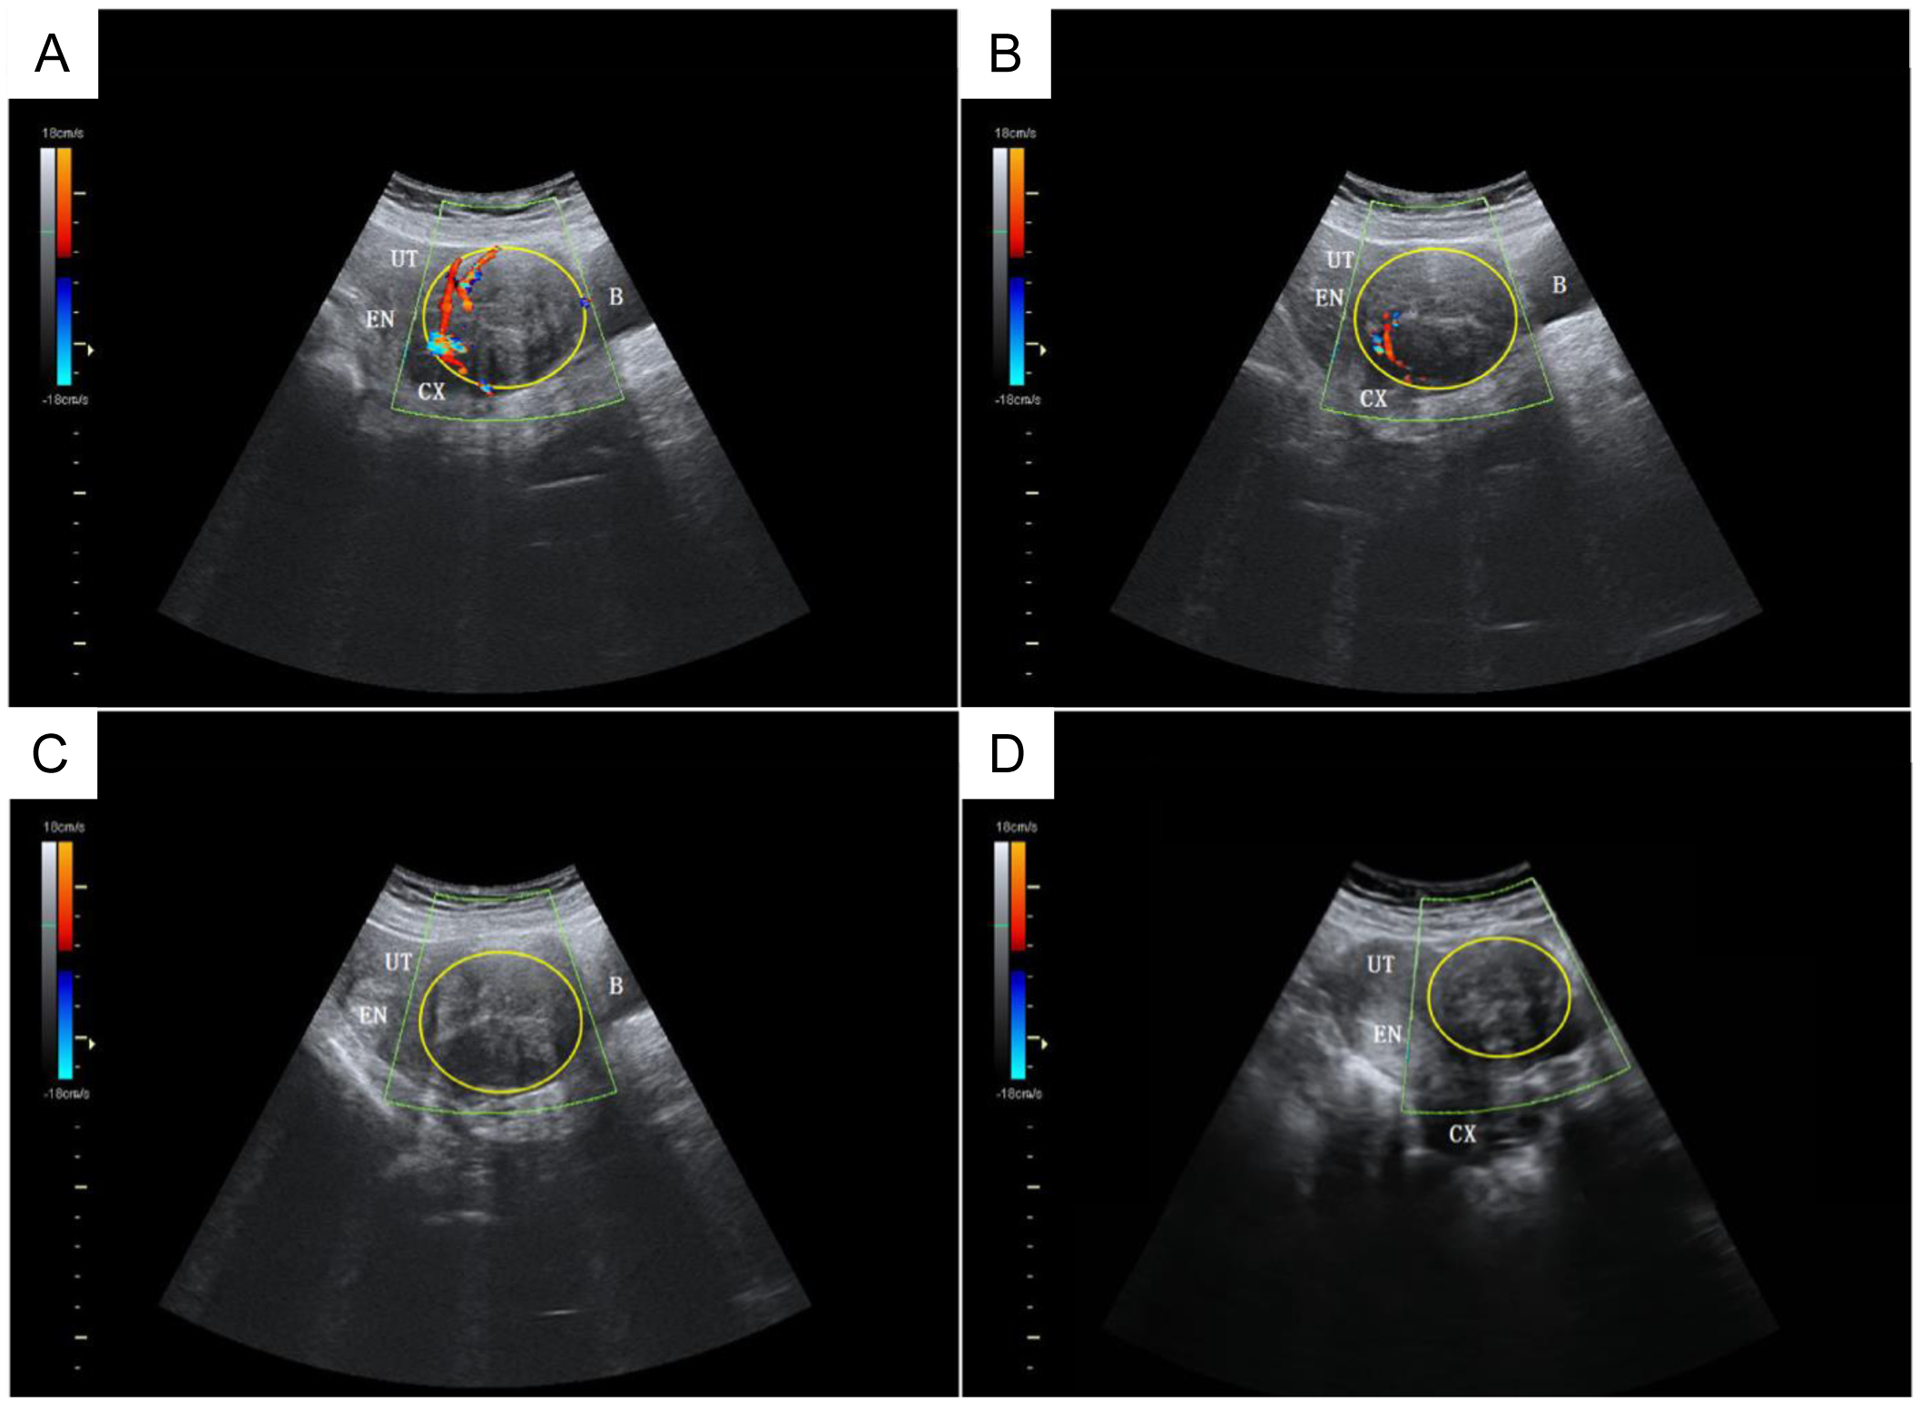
Color Doppler ultrasound imaging pre, peri, and postthermal HIFU ablation. b-mode ultrasound (US) images with color Doppler flow imaging (CDFI) from a 44-y-old patient before and after treatment with thermal HIFU. (a) Pretreatment image shows the vascularity of the fibroid for treatment. (b) Intraoperative assessment result shows that the targeted vessel in the treatment cell was not detected by CDFI and Power Doppler Imaging (PDI). (c) Posttreatment image shows the whole vascularity was not detected by CDFI and PDI. (d) US image with CDFI acquired 3 months after treatment shows fibroid shrinkage. The yellow ring indicates fibroid. B = bladder; CX = cervix; EN = endometrium; UT = uterine. Figure adapted from [18].
